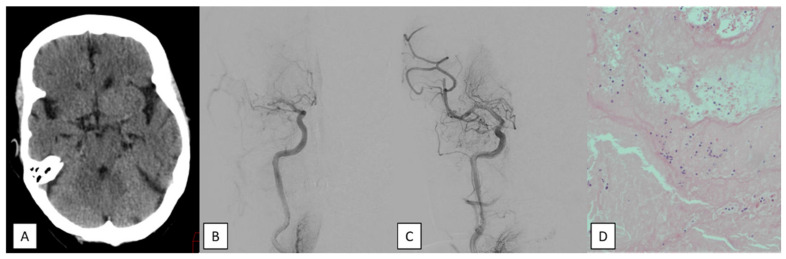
An 84-year-old female that was admitted with acute stroke with a NIHSS score of 19 points due to right M1 occlusion. (A) A non-contrast head CT that does not show a hyperdense vessel sign in the occluded right middle cerebral artery. (B) Digital subtraction angiography confirmed the right M1 occlusion. (C) Digital subtraction angiography after the forth pass with a stent retriever and stent–aspiration combination showing unsuccessful recanalization of the middle cerebral artery with clot migration into distal branches (TICI 2a). (D) HE20X predominance of platelets and fibrin, as well as scarce inflammatory cells and erithrocytes.
